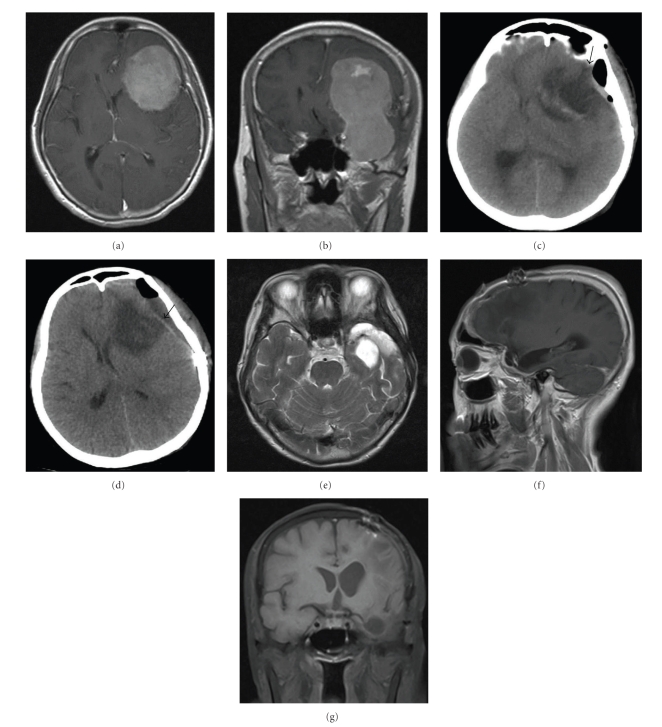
Imaging results for Case 2. (a)-(b) MRI showing sphenoid ridge meningioma. (c) CT revealing cyst formation in the tumor resection cavity 6 days after tumor resection. The arrow indicates the narrow roof region of the tumor resection cavity, where the “valve”-like structure is located. (d) Recommencement of effusion in the tumor cavity 1 week after the reoperation. The arrow indicates that the roof region of the tumor resection cavity became widened. (e)–(g) MRI showing disappearance of cyst formation in the tumor resection cavity 10 months after the reoperation.
